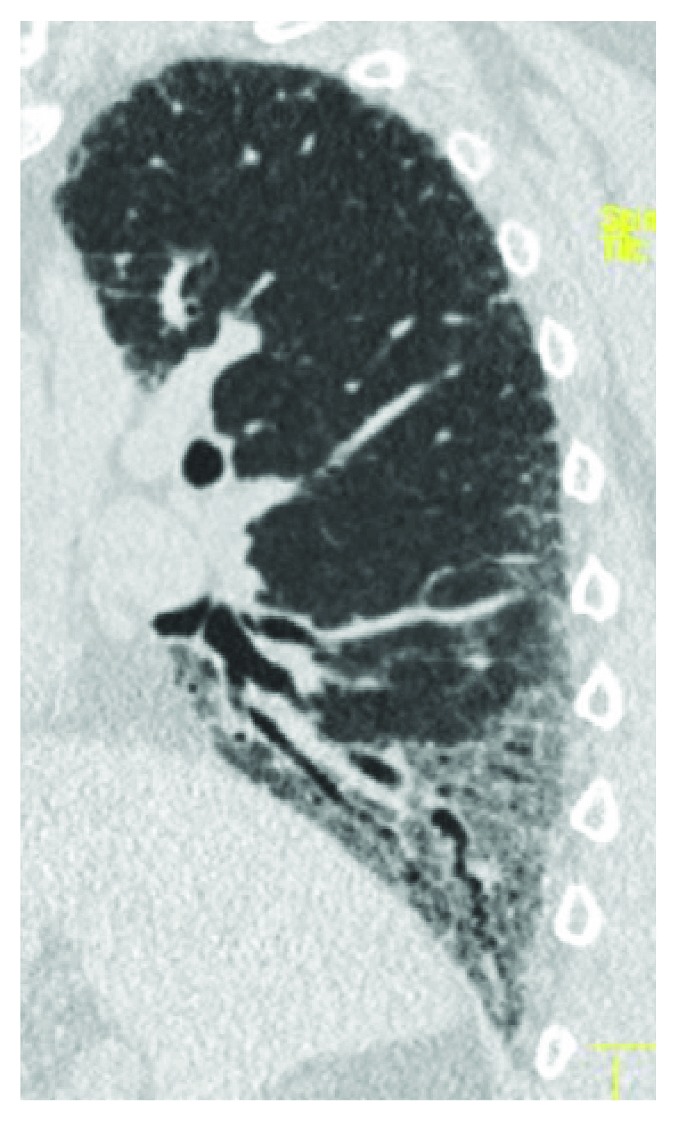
Sagittal HRCT of a 52-year-old male patient with CD shows lower bronchiectasis and bronchiolectasis, associated with diffuse basal parenchymal opacities (ground glass pattern) and interstitial septal thickening.
